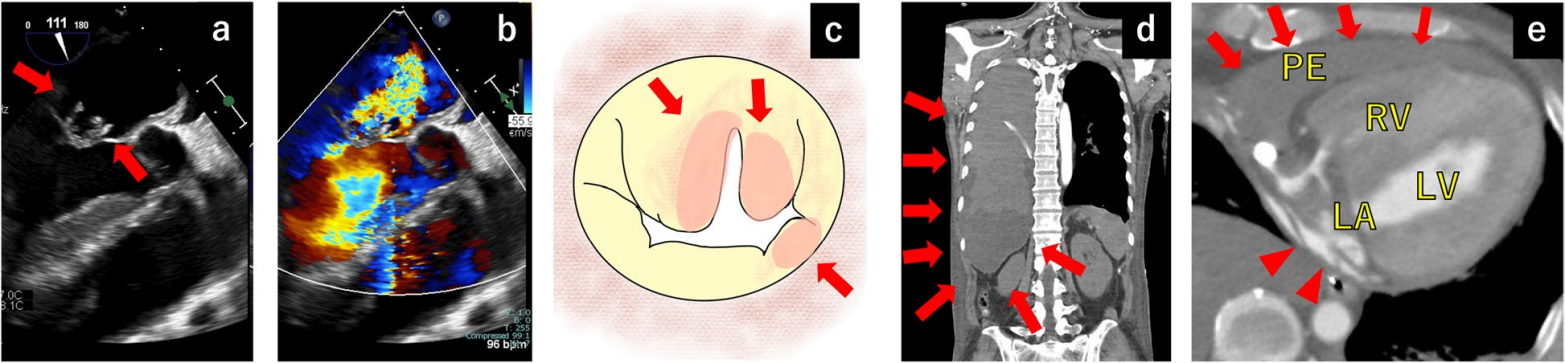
Preoperative transesophageal echocardiography, intraoperative findings, and postoperative contrast-enhanced computed tomography findings. a, b TEE shows severe mitral regurgitation with vegetation on both the anterior and posterior leaflets (A2, P3) (red arrows). c The vegetation was attached to the leaflets A2, A3, and P3, and there was also an abscess formation at the annulus, especially from P3 to the posterior commissure (red arrows). d and e Contrast-enhanced computed tomography reveals massive hematoma in the right thoracic cavity and pericardium (red arrows) and the extravasation from the left atrium (arrowheads). RV, right ventricle; LA, left atrium; LV, left ventricle; PE, pericardial effusion
